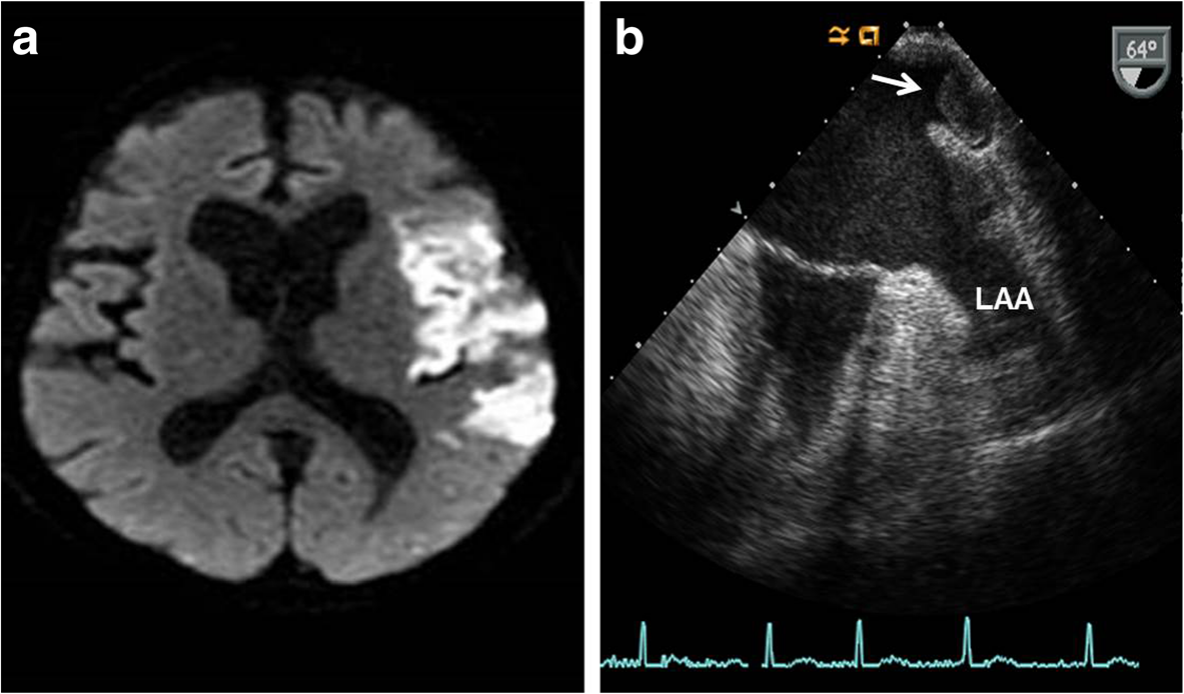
Brain magnetic resonance image demonstrating acute infarction in left middle cerebral aretry territory (a). Transesophageal echocardiography demonstrating about 1.6 × 1.4 cm sized thrombus within the remnant stump of the left superior pulmonary vein and no thrombi win left atrial appendage (LAA) (b)
